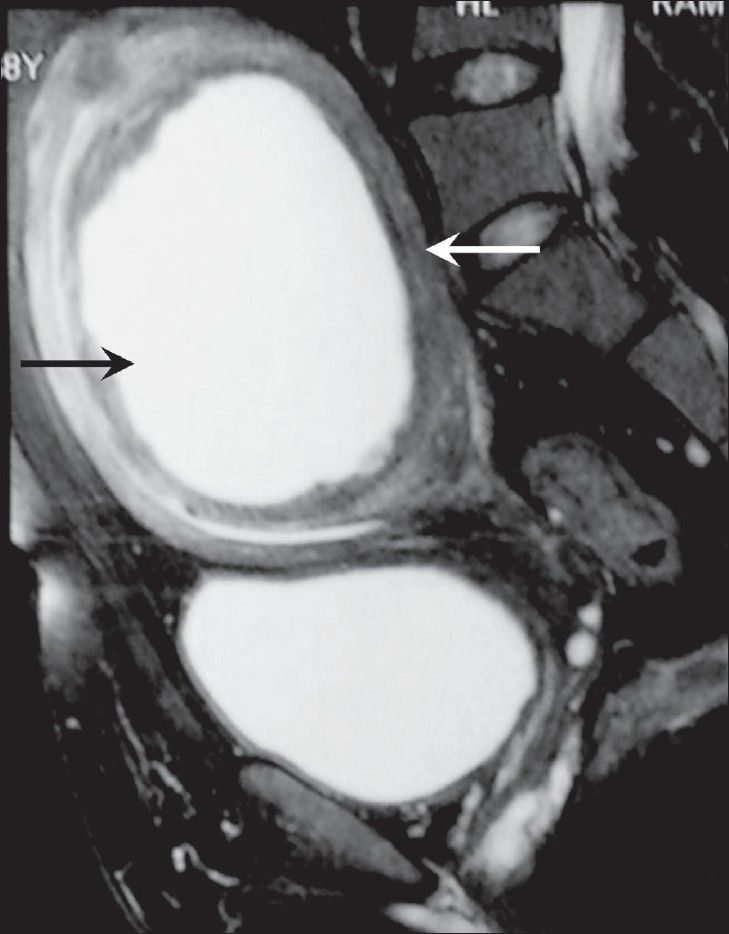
Coronal T2W fat-suppressed MRI shows a hypointense wall (white arrow) and hyperintense internal contents suggestive of cystic degeneration
